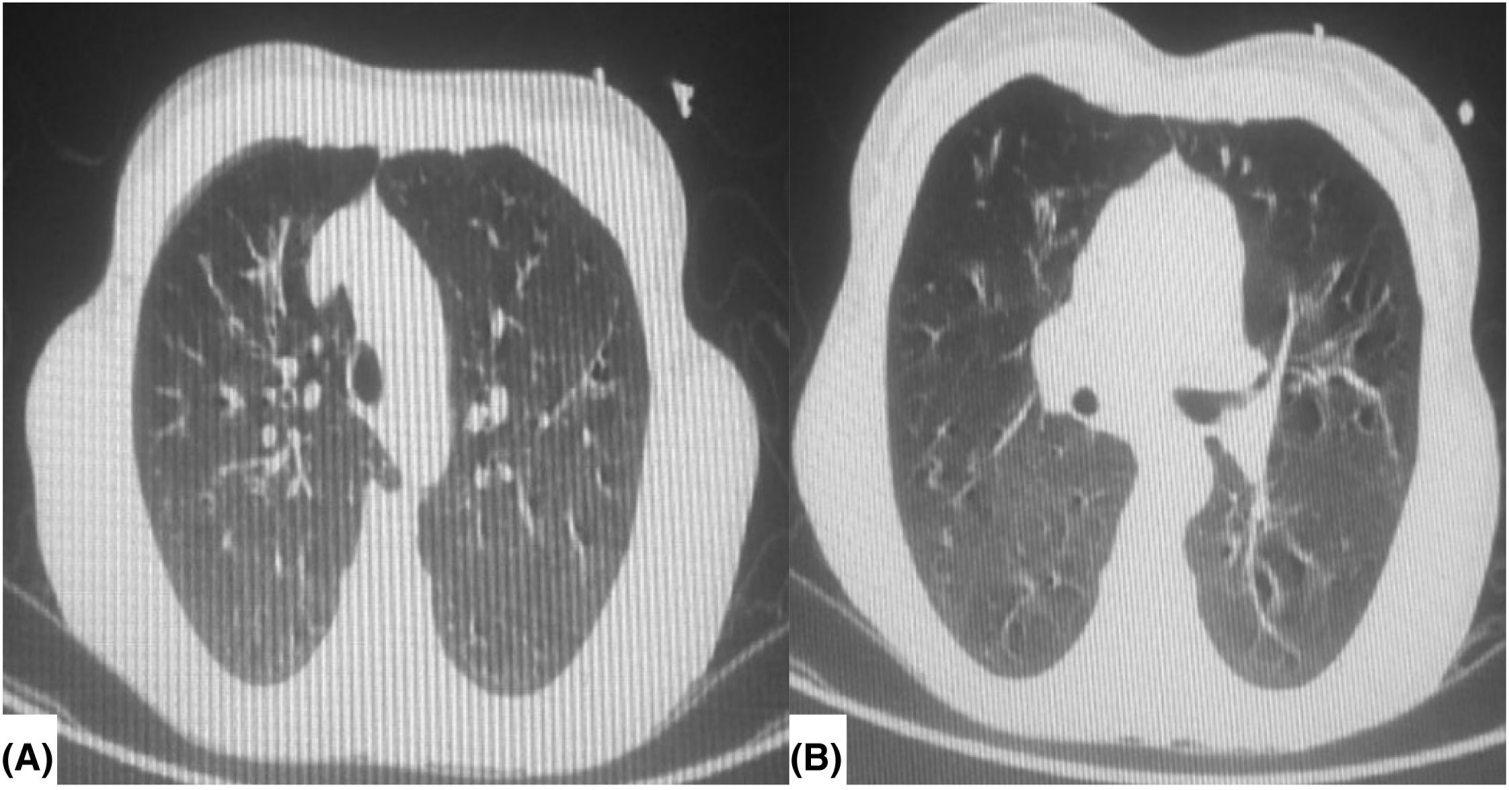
Chest CT transverse planes (A) and (B) show bilateral multifocal multilobular bronchiectasis with peribronchial thickening, consistent with acute bronchitis superimposed on chronic bronchitis.
